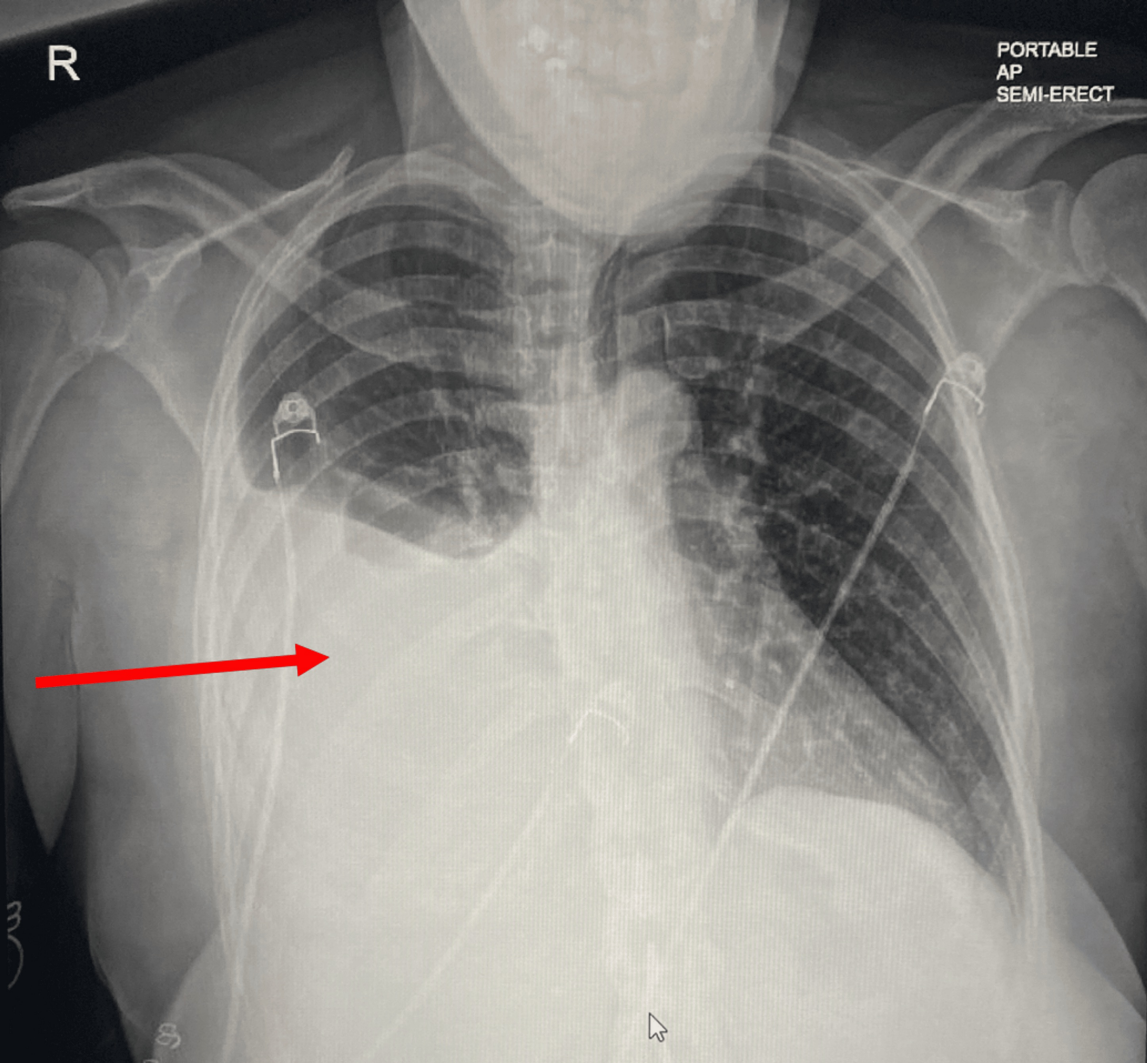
Chest Radiograph Showing a Large Right-Sided Pleural Effusion Before Thoracentesis Red arrow highlighting the area of interest.
